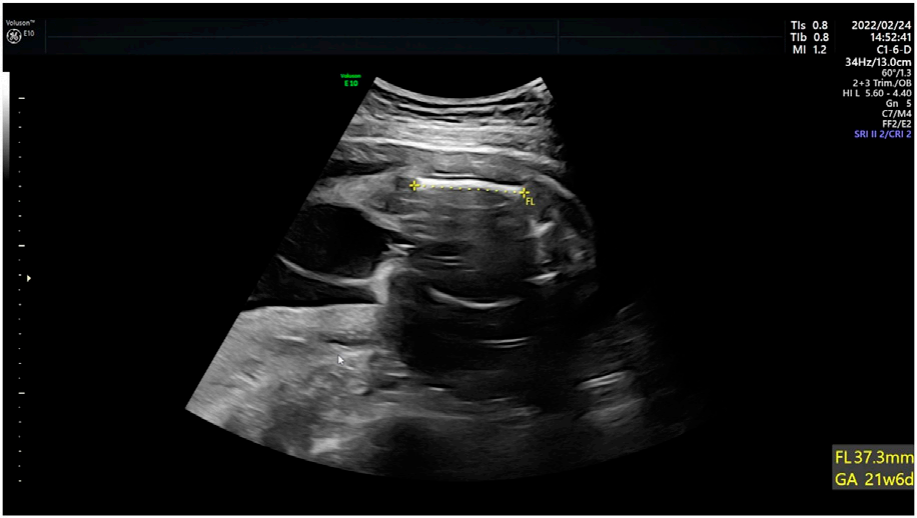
Antenatal ultrasound of foetus 4 The femur length was 3.7 cm and slightly bowing.
